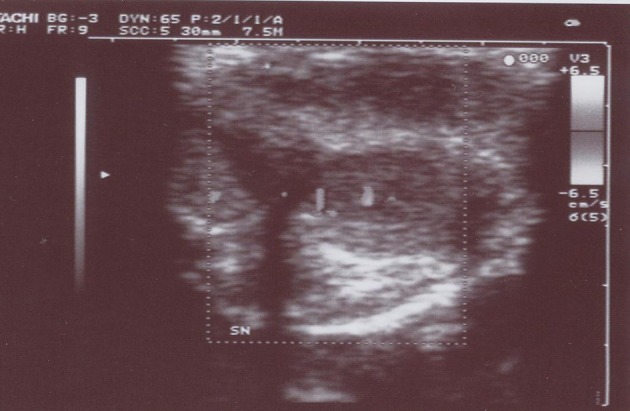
Ultrasonography of the lesion.
